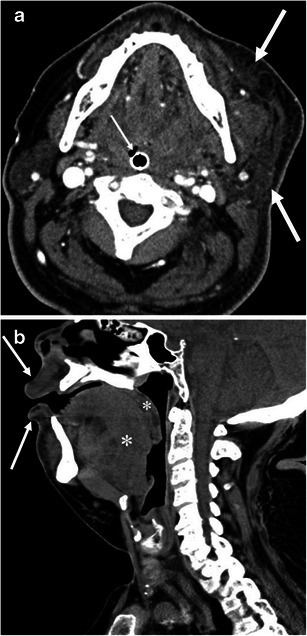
A 64-year-old male with ACE inhibitor-induced angioedema with airway obstruction. a The axial CE-CT image shows left hemifacial swelling with subcutaneous fat stranding (*large arrows*). The patient is status post placement of an endotracheal tube (*small arrow*). b One year later, the patient developed recurrent angioedema after tooth extraction. The sagittal reformatted image of the unenhanced CT shows marked swelling of the tongue base and soft palate (*asterisks*). Also noted is swelling of the lips (*arrows*)
